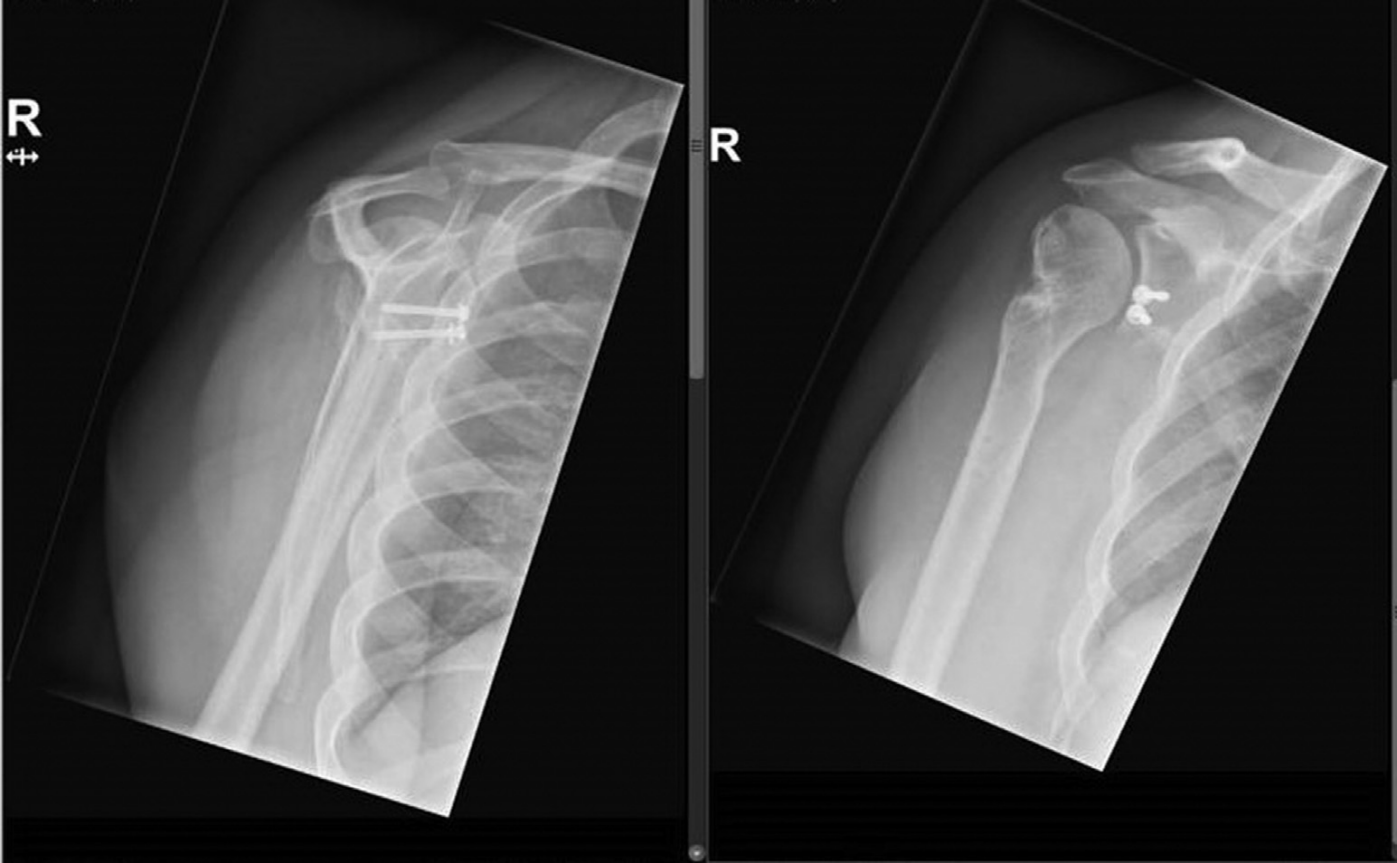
Postoperative radiographs after the removal of the humeral plate and screw fixation, showing an adequate position of the humeral head and the two lag screws of the Latarjet procedure.
